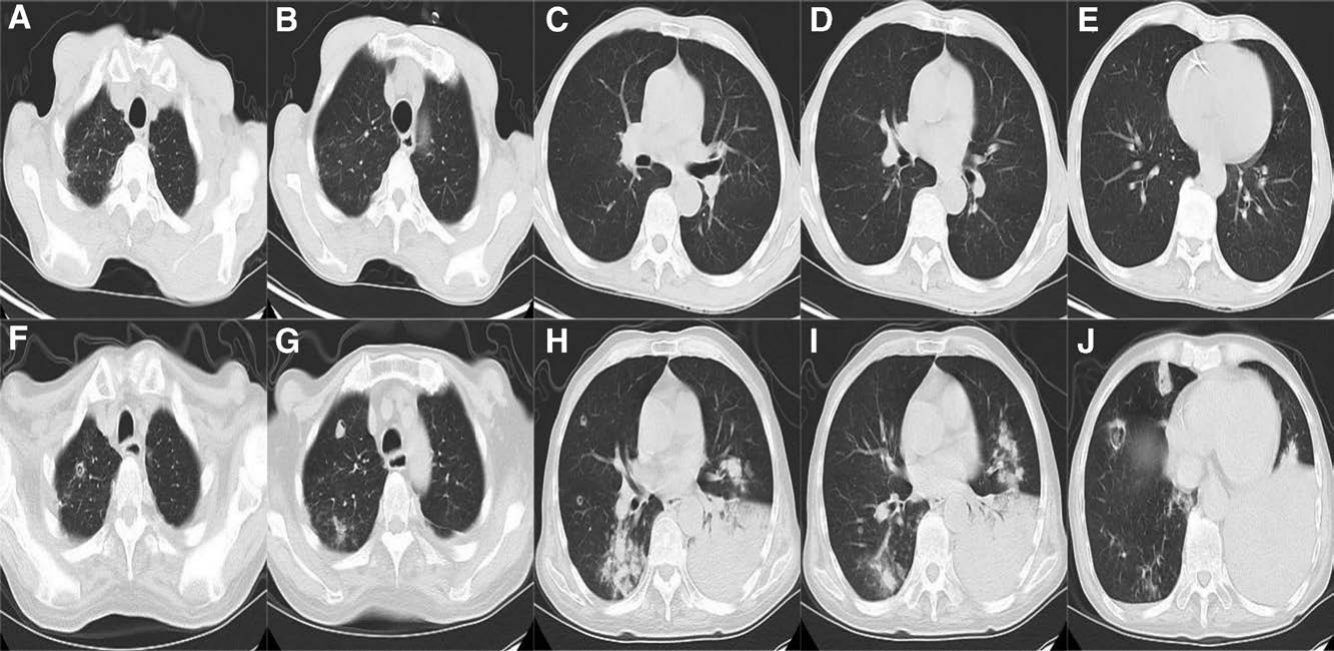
Patient lung computed tomography results. (A–E) Images were taken in June 2023. (F–J) Images were taken in November 2023.
